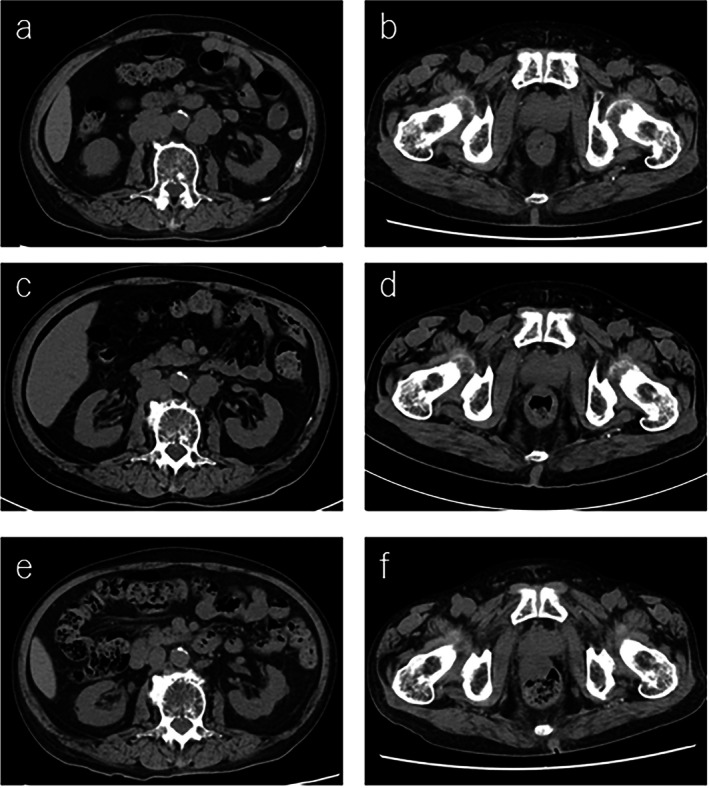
Images of abdominal contrast–enhanced computed tomography before (a, b), 1 month after (c, d), and 3 months after (e, f) the start of pembrolizumab treatment. (a, c, e) Images show a remarkable reduction in the size of the para‐aortic lymph node metastasis despite being taken 2 months after discontinuing pembrolizumab. (b, d, f) Images show tumor growth in the prostate region 2 months after pembrolizumab discontinuation.
